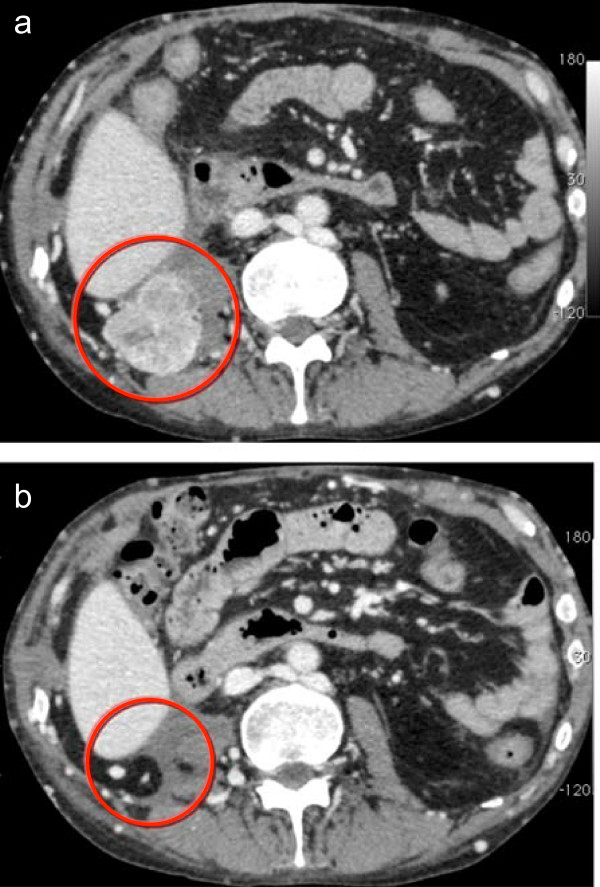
Postoperative computed tomography scan. A complete response was achieved after the 7^th^ course of sunitinib therapy for local relapse. Four months after surgery, a computed tomography scan showed that the tumor had increased to 45 mm (a). The administration of sunitinib was started. After 9 months of therapy, a complete response was achieved (b). The red circle indicates the local recurrent tumor.
